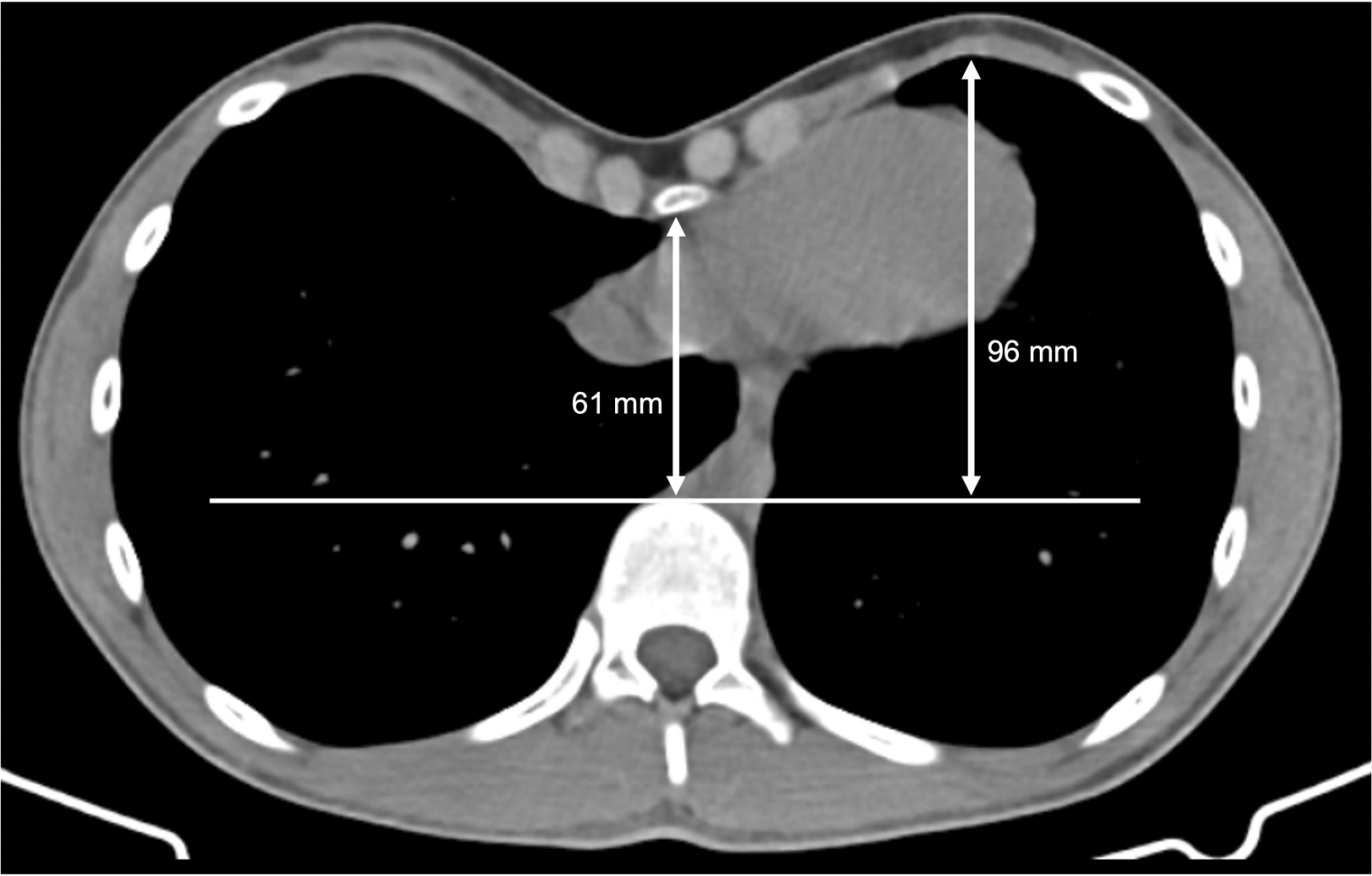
Preoperative thoracic computed tomography scan: axial image with correction index measures.
